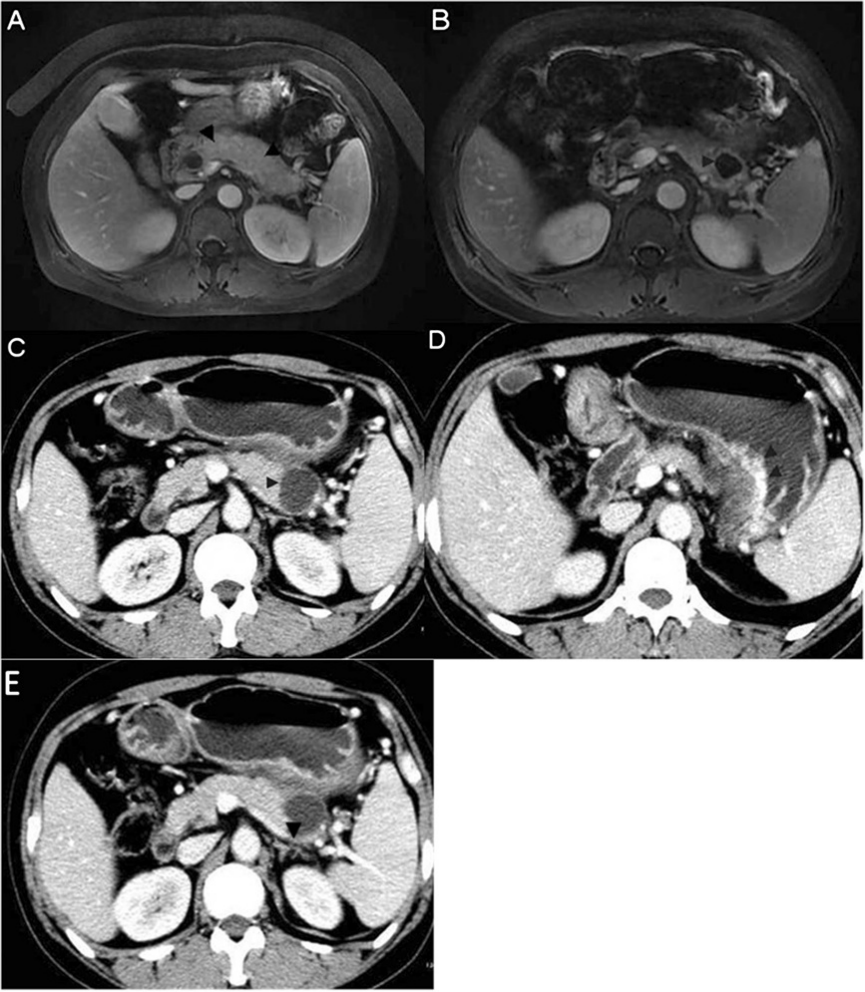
Diagnostic magnetic resonance imaging (MRI) and computed tomography (CT). (A) (MRI) diffuse enlargement of pancreas (arrowhead), (B) (MRI) cystic mass in tail of pancreas (arrowhead), (C) (CT) obviously enlarged cystic mass in tail of pancreas (arrowhead), (D) (CT) varices of gastric body (arrowhead), (E) (CT) thrombosis in splenic vein (arrowhead).
